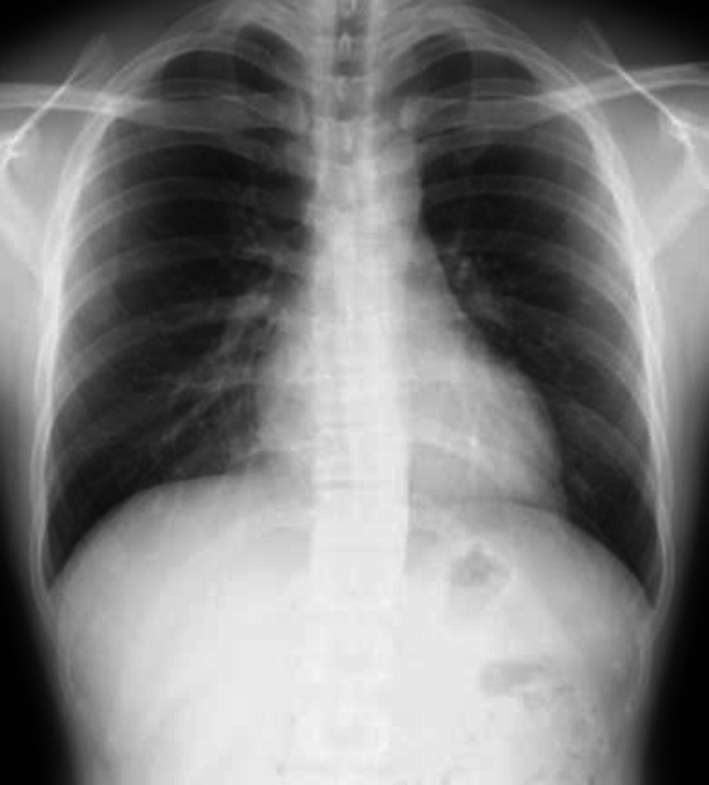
Postoperative chest radiograph 1 year after surgery
